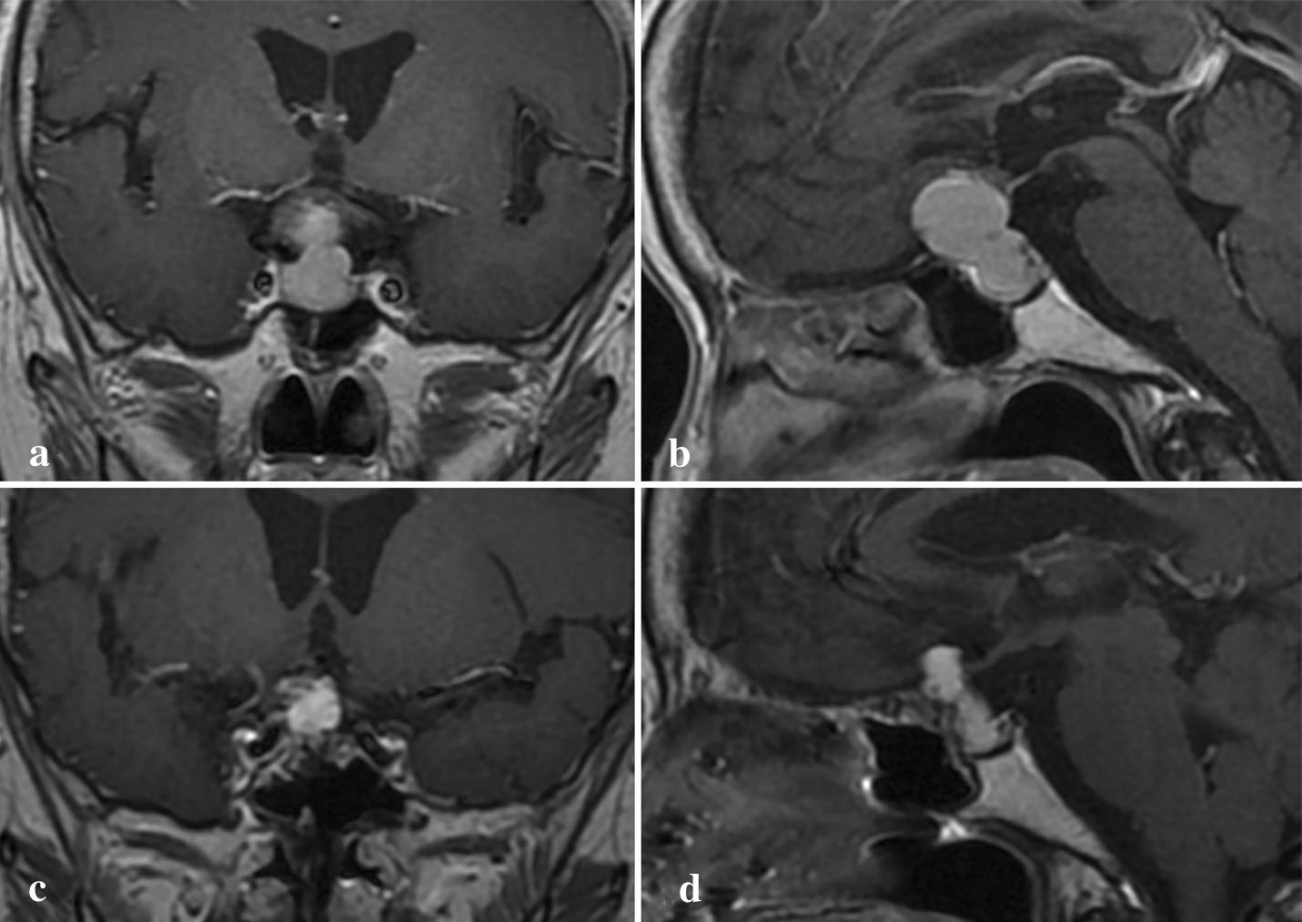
Case 2. a Preoperative coronal T1-weighted MR image with gadolinium revealing a dumbbell-shaped cystic lesion extending from the sella turcica to suprasellar and cistern. b Preoperative sagittal T1-weighted MR image with gadolinium revealing the cyst compressed optic chiasm upwards. Cyst was almost collapsed after the operation (c, d)
